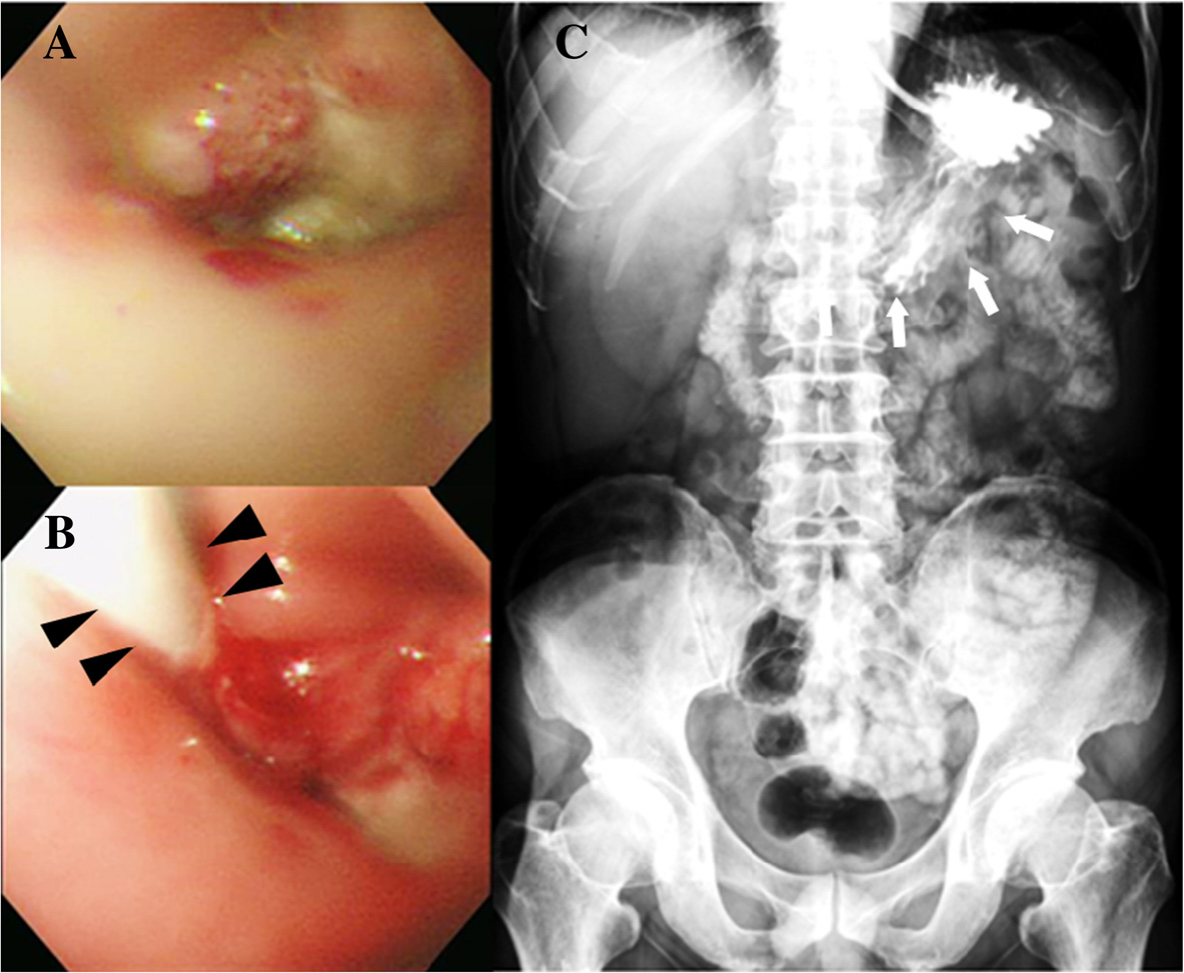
Representative images before and after endoscopy-assisted nasogastric tube placement (a) Esophagogastroduodenoscopy demonstrating nearly total obstruction of the esophagus by a tumor. b NG tube (black arrowhead) passed through the site of the obstruction. c Abdominal plain-film radiograph showing that the feeding tube is in the stomach (white arrow); reconfirmed using contrast medium
